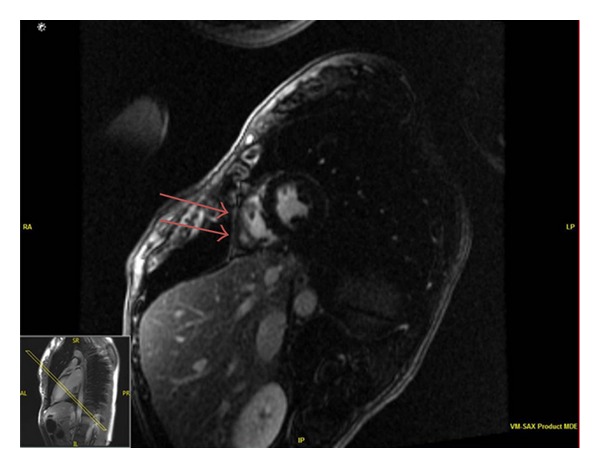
Pericardial enhancement of the right ventricular free wall on cardiac MRI consistent with acute pericarditis.
